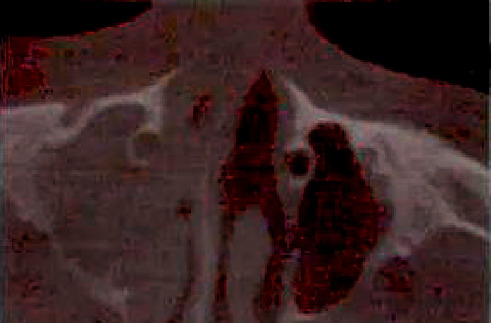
Traditional segmentation of the CBCT image of apical periodontitis.
